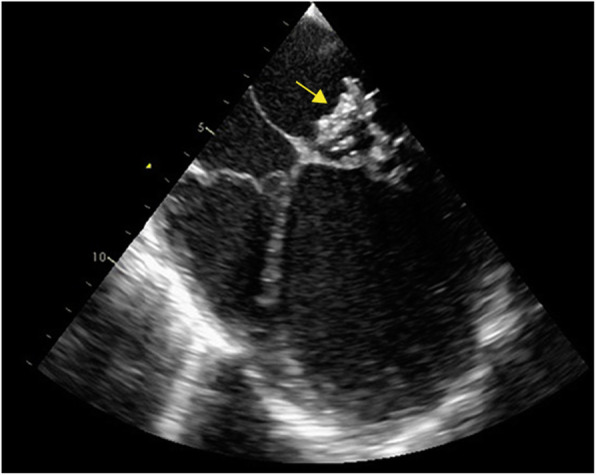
Large mitral vegetation (yellow arrow) in the transesophageal echocardiography
